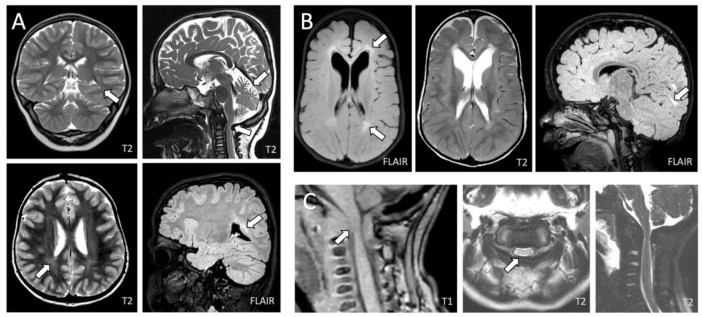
Brain and cervical spine abnormalities found in patients with MLII and ML intermediate via magnet resonance imaging (T2-/T1-weighted and FLAIR sequences). (A) Atrophy of the top part of the cerebellum and vermis, cervical spinal canal stenosis, and white matter changes in an 11.2-year-old ML intermediate female (patient 9). (B) White matter signal changes in a female 2.4-year-old (patient 6; pictures in transverse plane) and a male 3.2-year-old MLII patient (patient 5; sagittal plane). (C) Cervical spinal canal stenosis in a 3.3-year-old MLII male (patient 8).
